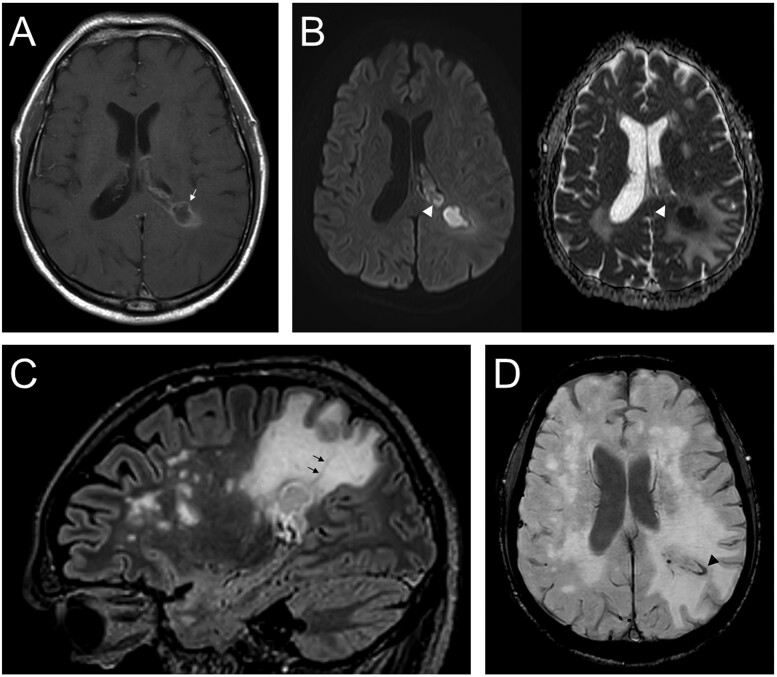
MRI of brain abscess with *Nocardia* and *Toxoplasma* coinfection. (A) Axial T1-weighted image demonstrates a peripherally enhancing left parietal lesion with central T1 hypointense complex fluid contents (arrow). (B) On diffusion-weighted imaging (left) and the corresponding apparent diffusion coefficient map (right), the lesion exhibits central diffusion restriction extending into the ventricle (arrowhead) indicating internal pus and pyogenic ventriculitis. (C) Sagittal FLAIR acquisitions confirm communication between 2 multilobulated components (arrows). (D) There is substantial hypointense signal dropout on susceptibility-weighted imaging, indicating intralesional hemorrhage (arrowhead).
